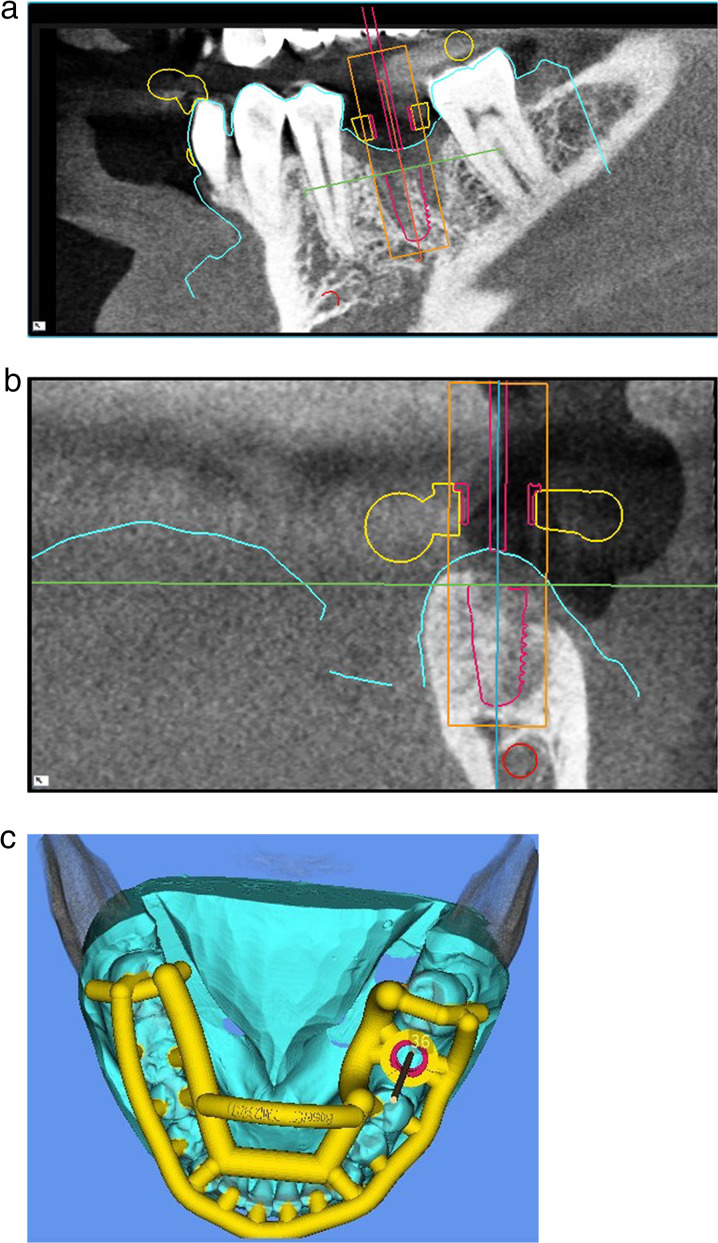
a–c Virtual implant planning (SMOP, Swissmeda AG, Baar, Switzerland) in region 36. The blue outlines represent the STL data of the intraoral scan which was matched over the CBCT. c Shows the design of the surgical template
